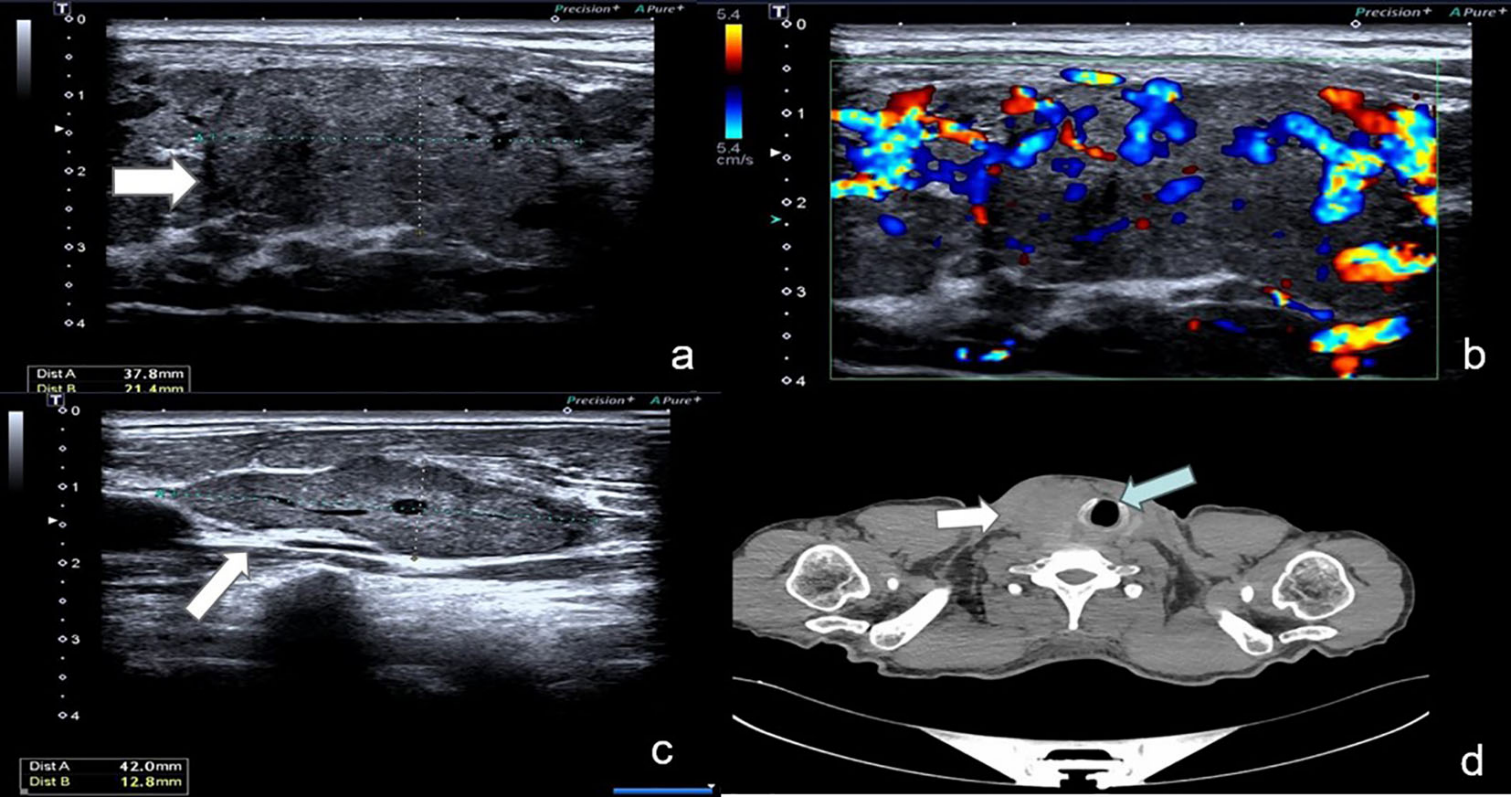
Ultrasound and CT of the thyroid gland and neck. (A, B) Multiple hypoechoic nodules were identified in the right lobe of the thyroid gland, some of which were partially fused, forming a mass with a maximum dimension of approximately 38*21mm. The nodules exhibited irregular morphology and were associated with abundant blood flow signals. (C) Multiple enlarged lymph nodes were detected in the right cervical region, the largest of which was about 42*13mm and contained abundant blood flow signals. (D) CT shows that the right lobe of the thyroid gland exhibited enlargement, accompanied by the presence of a slightly hypodense mass measuring approximately 4.3*4.9 cm. The surrounding fat interstitial space appeared blurred, and the lesion showed indistinct demarcation from the adjacent trachea and esophagus, resulting in leftward displacement of the trachea.
